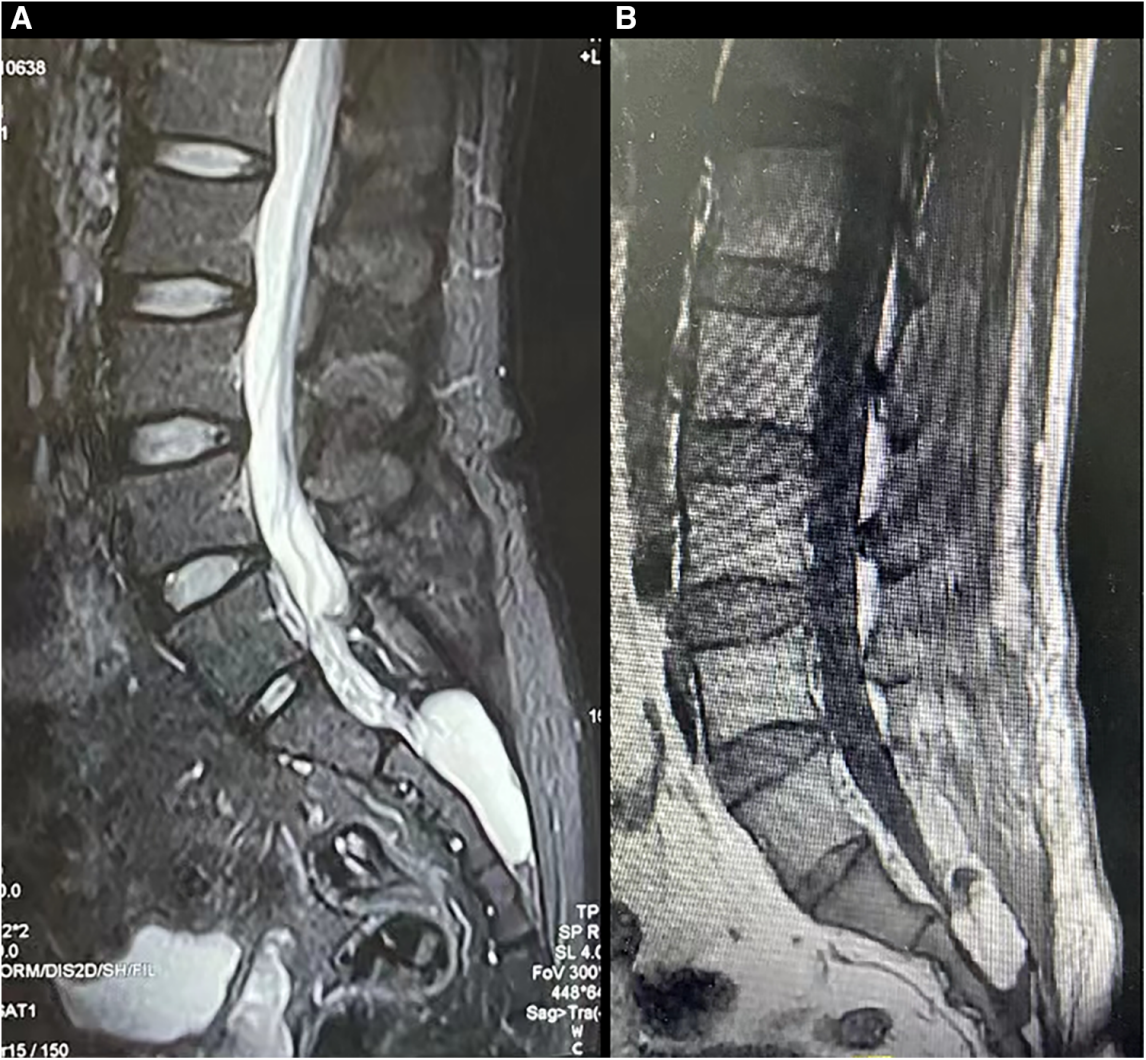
The MR images of a patient with a symptomatic Tarlov cyst before and after surgery were presented (case 2). Before surgery, T2-weighted sequences (A) revealed the presence of a Tarlov cyst filled with cerebrospinal fluid in the sacral canal. Following surgery, the original space occupied by the Tarlov cyst was replaced by fat tissue (B).
